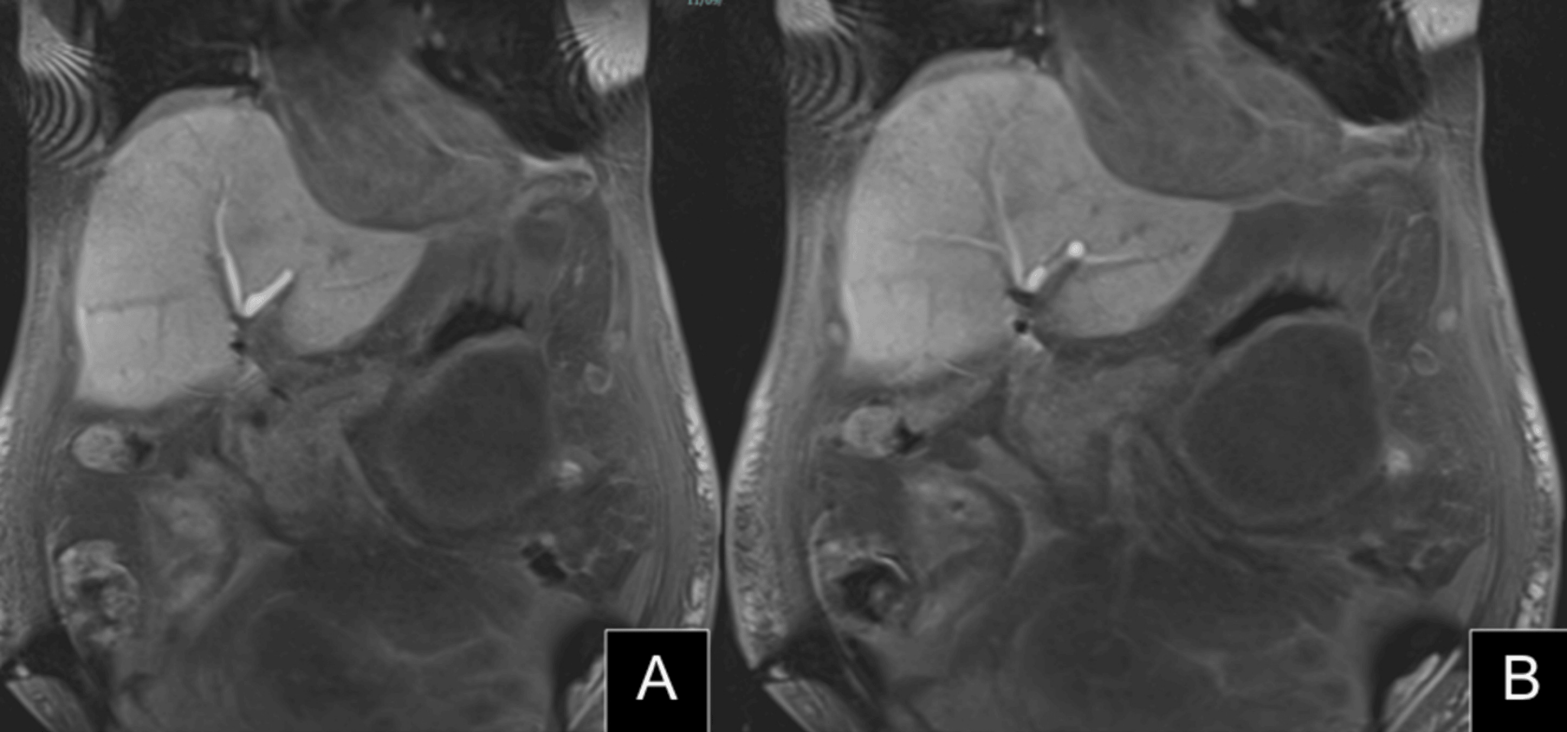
Representative coronal images from the initial MRI scan (A) Redemonstration of previous fluid collections. (B) A metallic artifact is seen at the junction of the right and left hepatic ducts with the inability to visualize the common hepatic or common bile duct.
